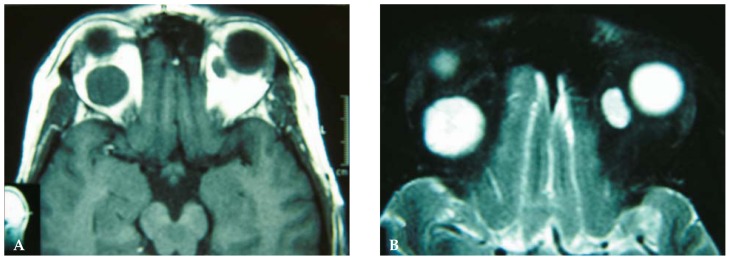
(A) T1-weighted magnetic resonance imaging shows a well-defined mass of intermediate density relative to brain tissue and isointense relative to muscle in the orbital cavity on both sides. (B) T2-weighted image after gadolinium injection shows marked uniform enhancement of the masses.
